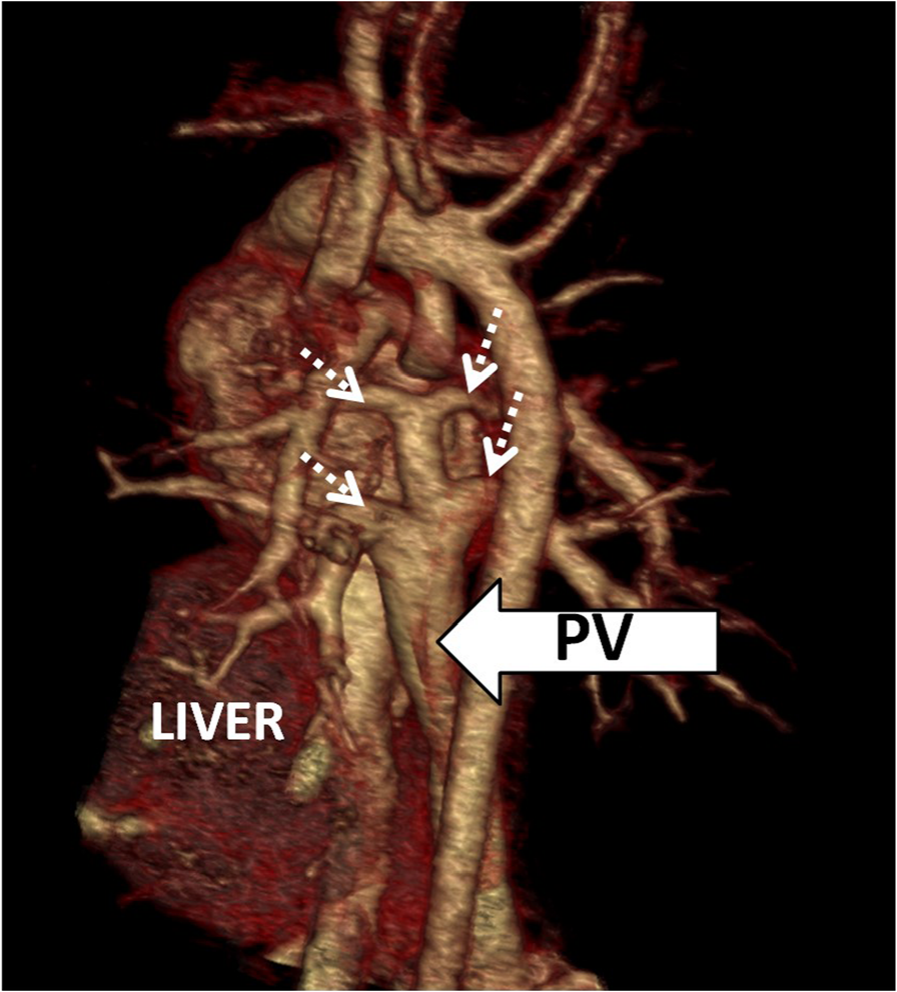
Cardiac CT of a 1 year old univentricular left ventricle patient showing infracardiac type total anomalous pulmonary venous returns (dashed arrows) which draining into portal vein (arrow). (PV; portal vein)
